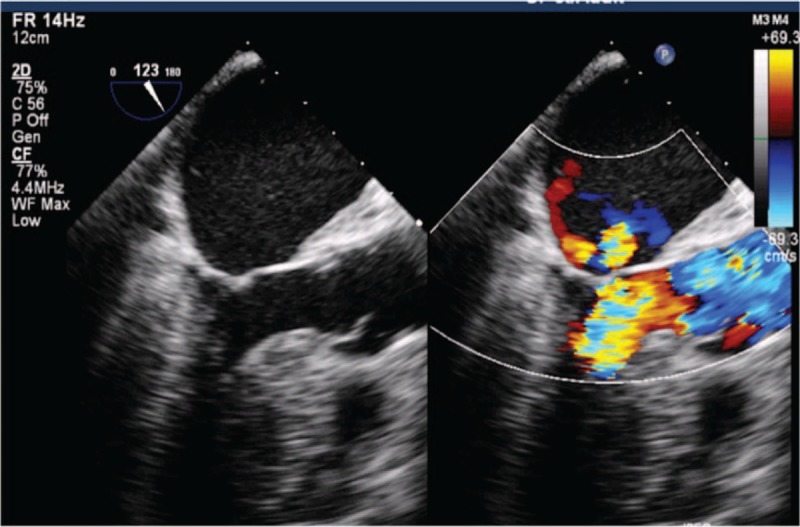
The postoperative transesophageal echocardiogram showed that the LV tumor was resected completely.
